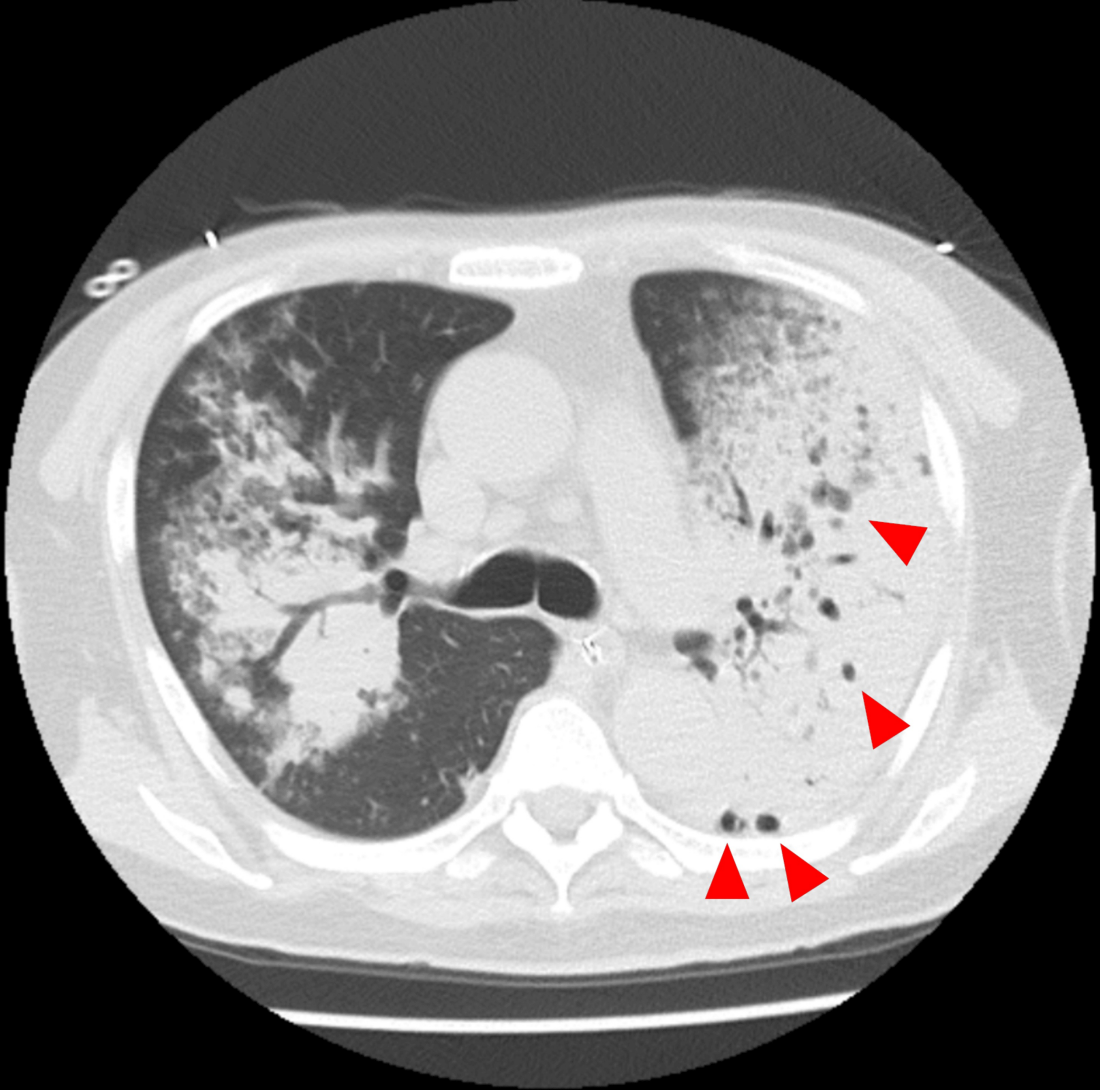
Contrast-enhanced chest CT showing bilateral consolidations and cavitary lesions (red arrowheads) predominantly in the left lung CT: computed tomography
